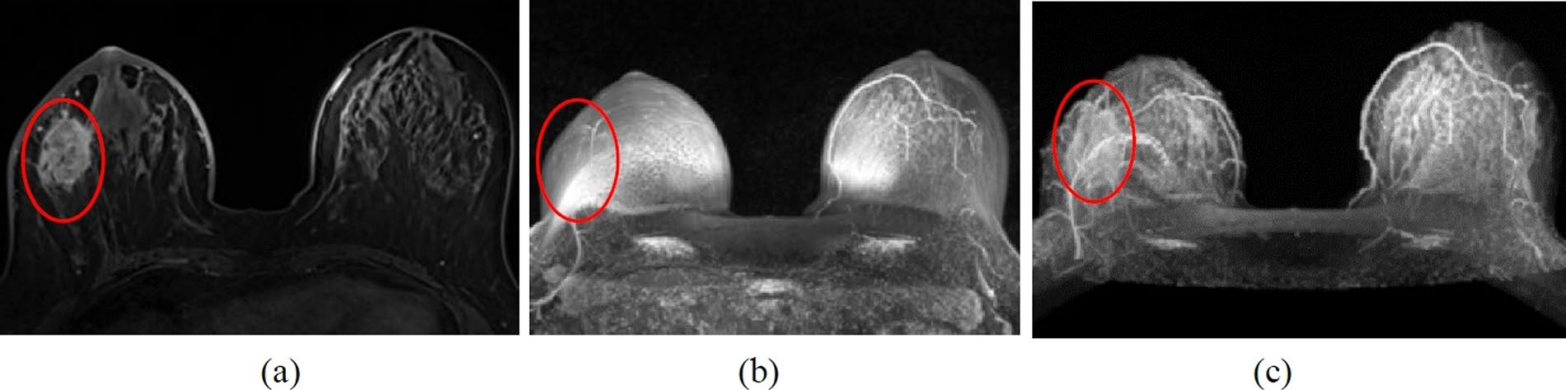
(a) Single DCE-MRI image. (b) 3D imaging of the original image. (c) 3D imaging of skin manually removed from original image. It is very difficult to segment the skin of DCE-MRI because of the following reasons: (1) Each case with 144 DCE-MRI sequence images. (2) There may be blood vessels connected to the mammary glands around the skin in each DCE-MRI (Fig. 2(a)). (3) The skin position and thickness are different in each image (Fig. 2(b)). The aforementioned skin characteristics can be overcome in the skin segmentation algorithm proposed in this study.
